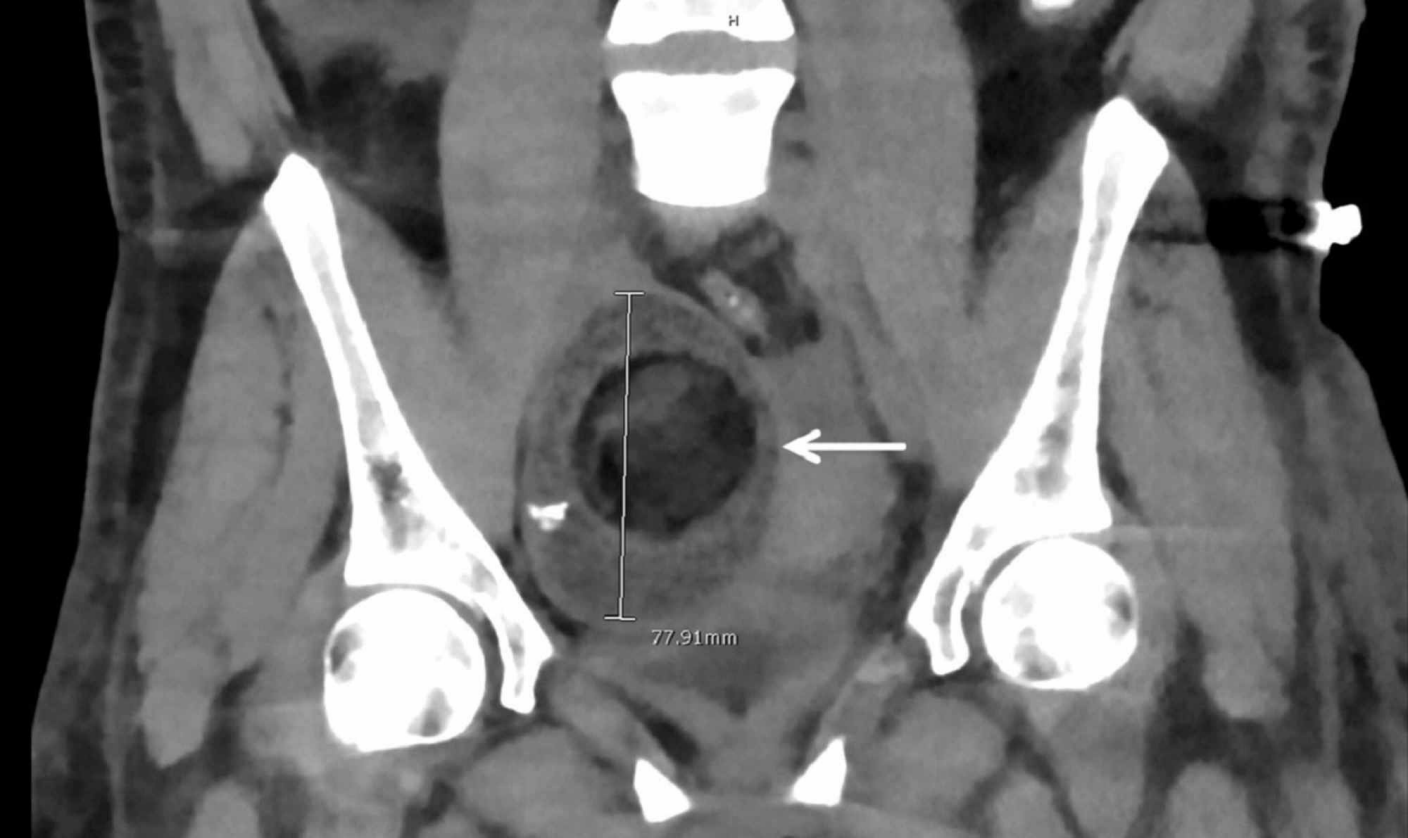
Computed tomography scan of the abdomen and pelvis showing the teratoma (arrow; coronal view).
